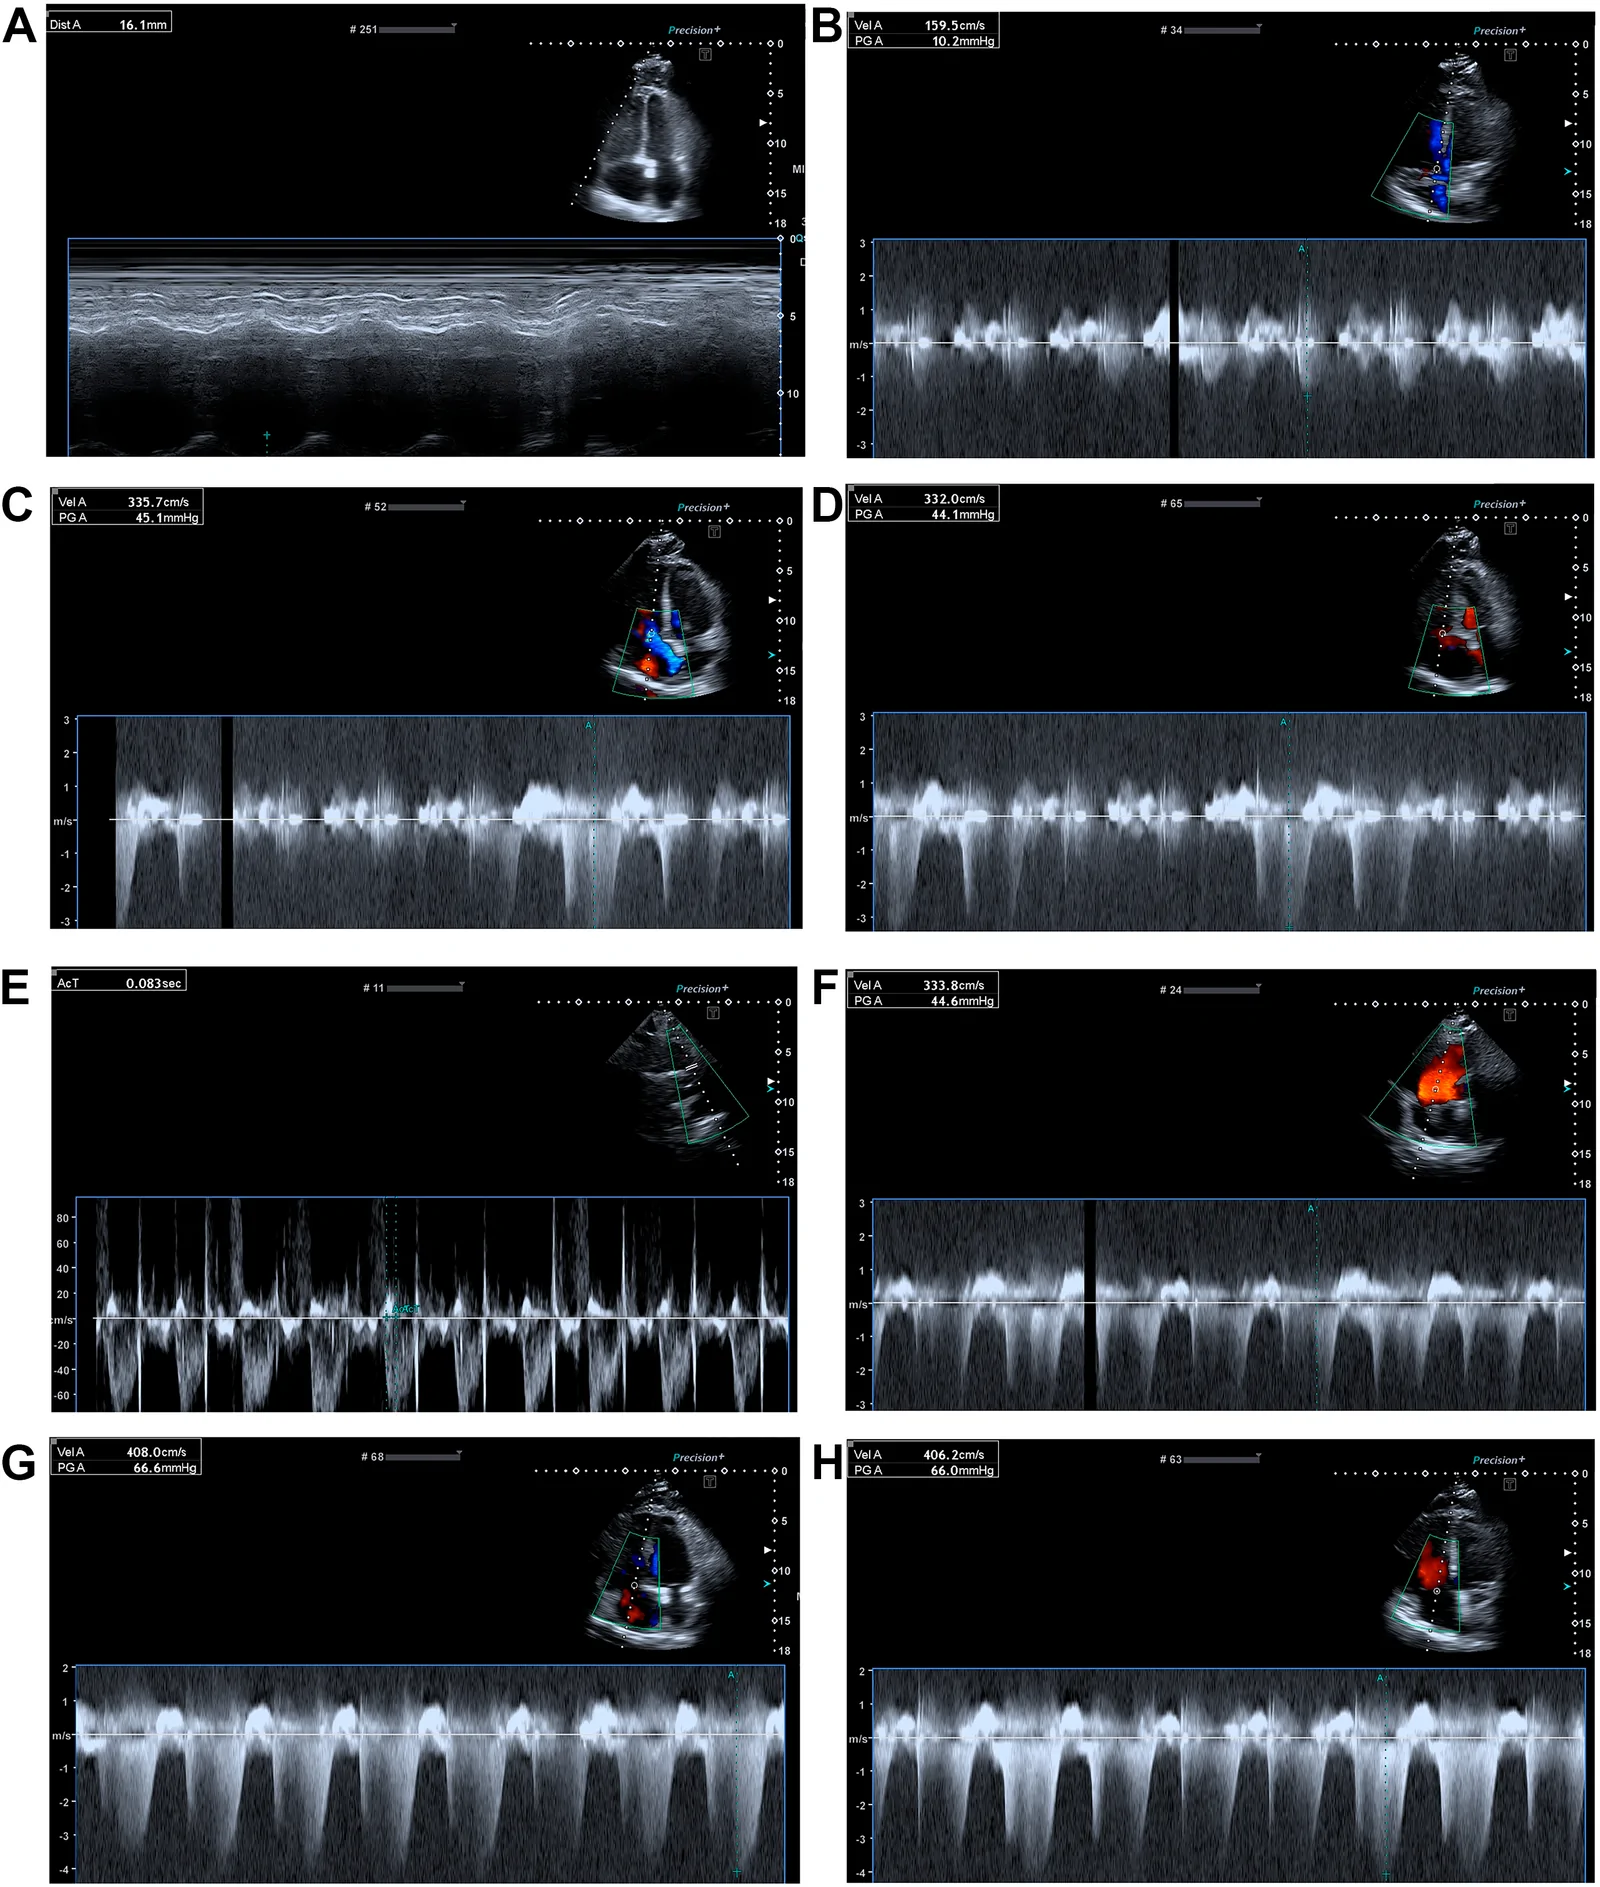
Transthoracic Echocardiography Demonstrating Right-Sided Cardiac Dysfunction and Pulmonary Pressure Overload (A) Apical 4-chamber view demonstrating marked right ventricular and right atrial dilatation with relative compression of the left ventricle, consistent with right ventricular pressure and volume overload. Interventricular septal flattening is noted, suggestive of increased right ventricular pressure. (B to D) Color Doppler imaging across the tricuspid valve demonstrates moderate to severe tricuspid regurgitation. Continuous-wave Doppler interrogation reveals a high-velocity regurgitant jet, indicating elevated right ventricular systolic pressure. (E) Pulsed-wave Doppler assessment of right ventricular outflow tract flow, demonstrating an altered systolic flow pattern consistent with findings suggestive of pulmonary hypertension. (F to H) Continuous-wave Doppler recordings showing increased tricuspid regurgitant jet velocity, supporting the presence of significant echocardiographic evidence suggestive of elevated pulmonary pressures and right ventricular strain, consistent with right-sided heart failure.
